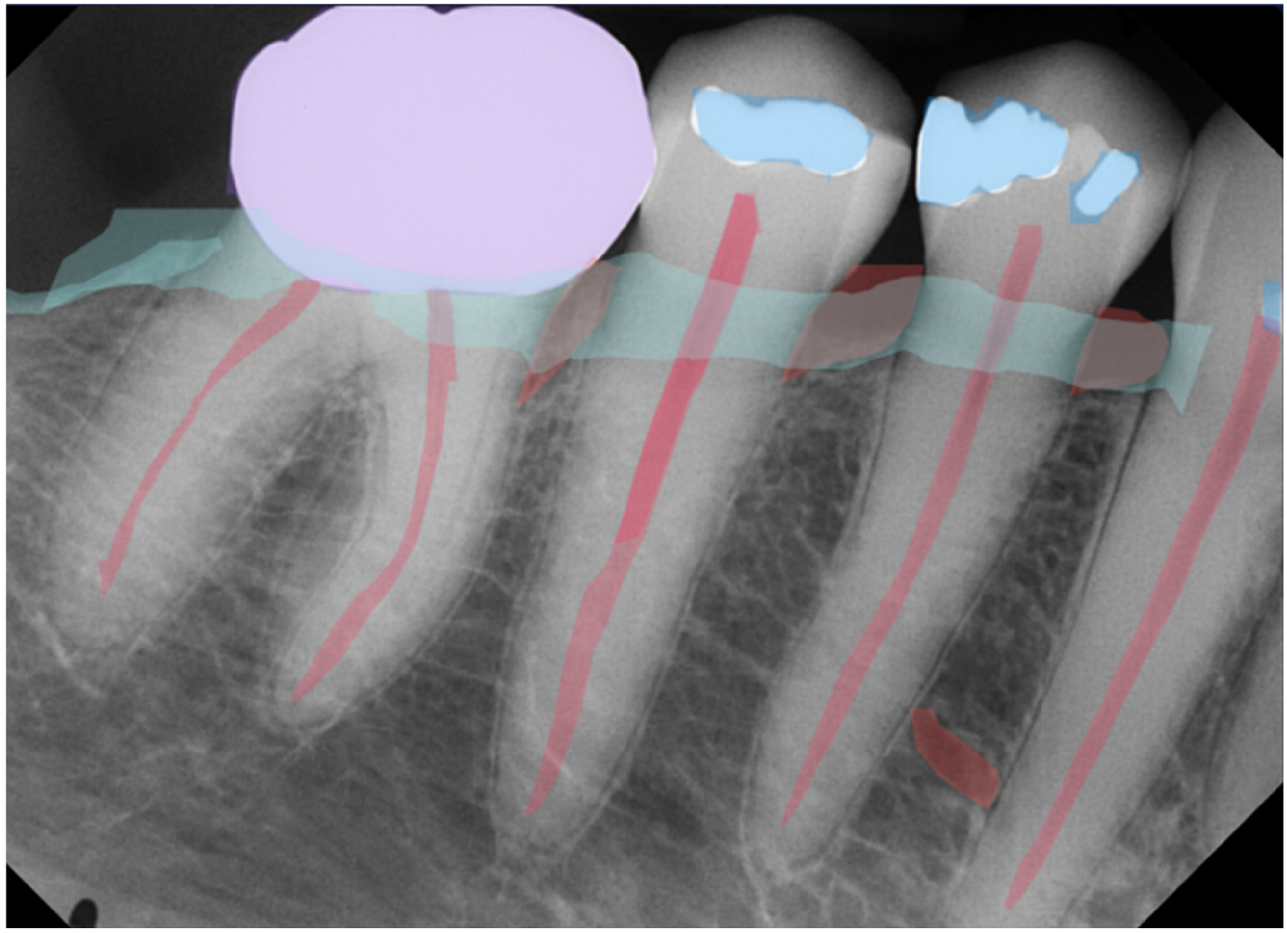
Restoration, crown, periodontal bone loss, and root canal segmentation as observed in periapical radiographs. (Courtesy—CranioCatch AI software).
